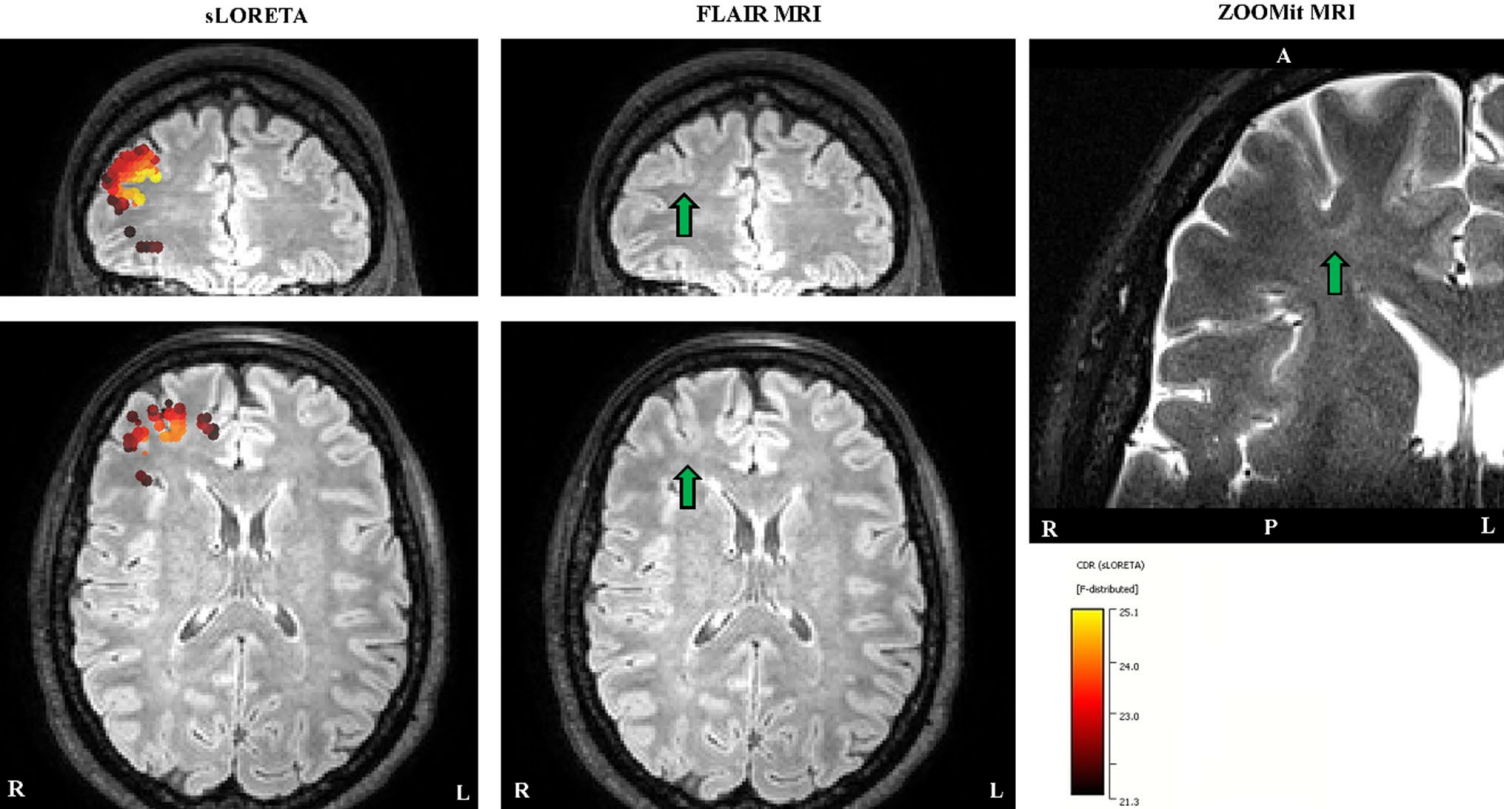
EMEG source localizations at −7 ms. In the left column the sLORETA results projected onto the FLAIR MRI are shown (only results obtained with a threshold of 85% for the maximum F-value are shown). In the middle column, the FLAIR image without the localizations is shown, in order to enable the identification of the FCDs (pointed to by *green arrows*). The right column shows the result of ZOOMit MRI with the *green arrow* indicating the FCD. *White letters* on the MRIs show the directions (*L* left, *R* right, *A* anterior, *P* posterior). (Color figure online)
